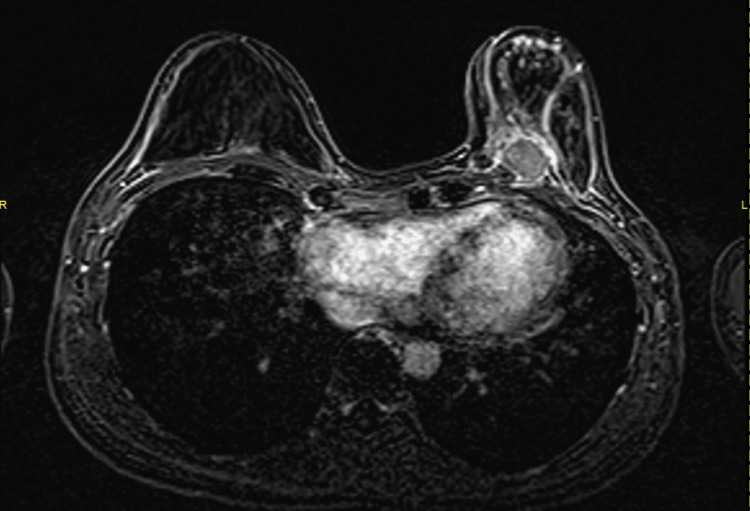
Dynamic contrast-enhanced MRI breasts in axial slice showing left 7 o'clock round rim-enhancing mass lesion with invasion of the underlying left pectoral muscle. No evidence of multifocality or multicentricity.
